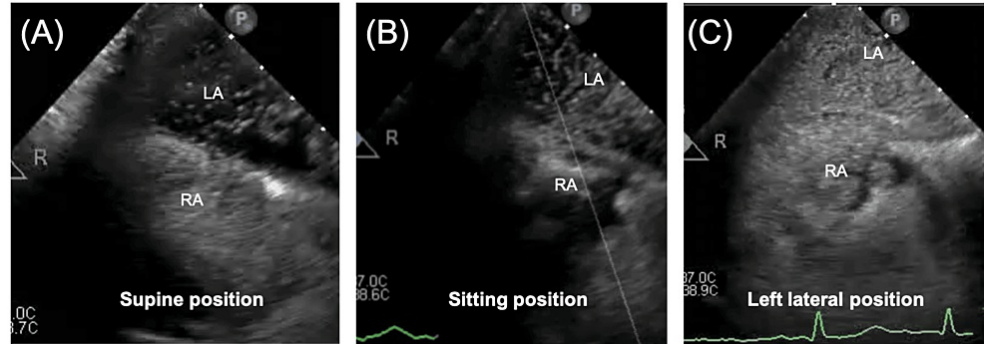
Transesophageal echocardiography maximal bubble study images for each positional change and lung perfusion scintigraphy. (A) Grade IV bubble inflow into the left atrium was observed. (B) The inflow of bubbles was increased compared to the supine position. (C) The entire left atrium was filled with bubbles, and more bubbles were observed than in other positions.
